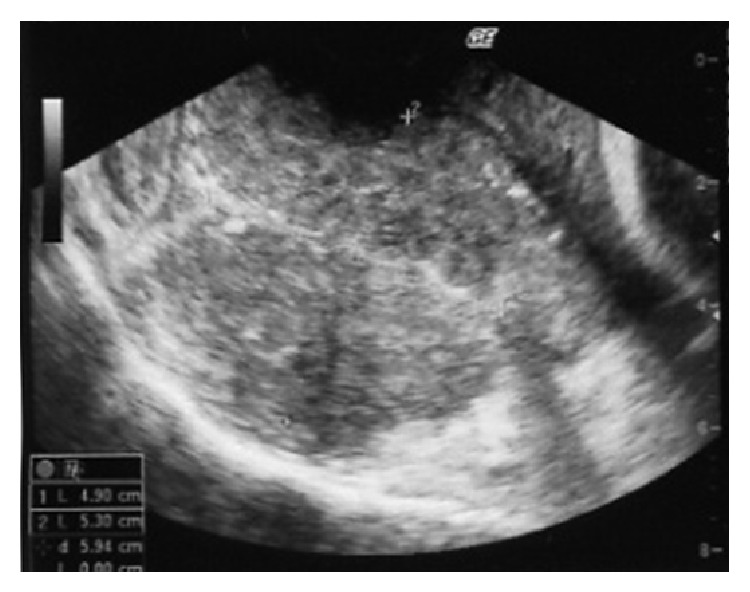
Vaginal ultrasonography demonstrated a 4.9 × 5.3 cm hypoechoic lesion behind the uterine cervix.
